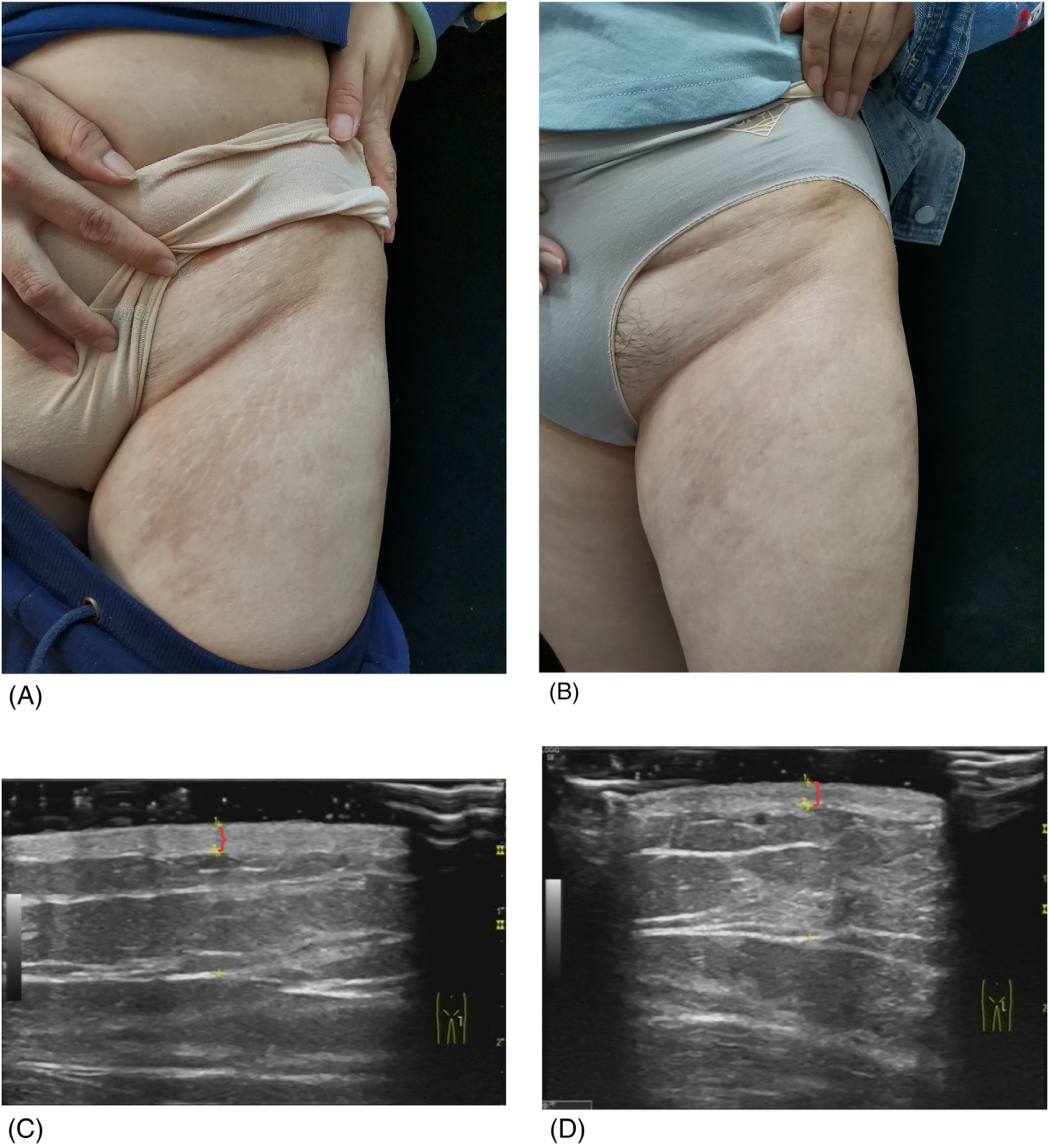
(A and B) Localized morphea lesions on the groin. The hardness, pigmentation, and atrophy of the skin lesions were significantly improved during the 6‐month treatment period. (C and D) Changes of skin thickness (the part indicated by the red bracket) before and after treatment under ultrasonography monitoring (skin thickness decreased from 0.21 cm to 0.18 cm).
